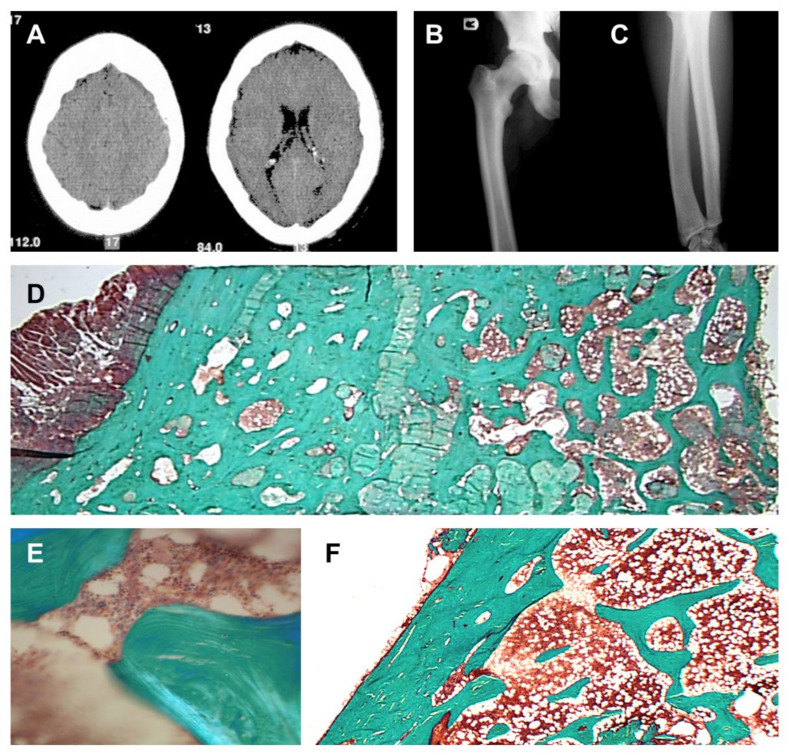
Bone phenotype in the proband. (A) CT scan of the patient’s skull, demonstrating a thickened calvarium. (B,C) Radiograph showing endosteal cortical hyperostosis of the femur (left), and radius and ulna (right). (D) Bone biopsy showing very high cancellous bone volume, no evidence of an increased osteoid volume, and the absence of active osteoblasts on the bone surface. Magnification 10× (insert (E), magnification 125×). (F) Bone biopsy of a healthy control demonstrating a clear distinction between the cortical and trabecular bone compartment, and normal bone volumes.
